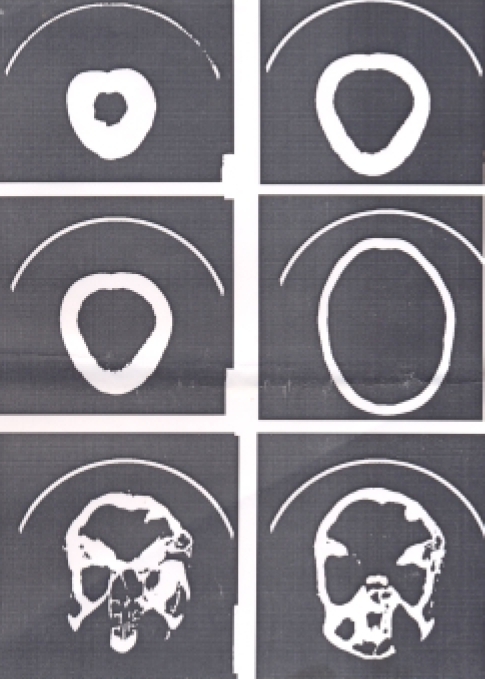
CT scan showing multiple subependymal nodules along the walls of the lateral ventricles. Cortical tubers are seen in the posterior fossa.
